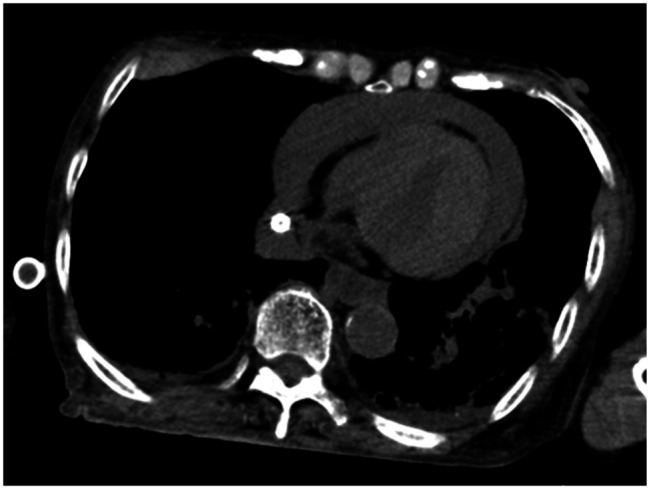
Axial chest computed tomography (CT) image on admission. Imaging revealed a prominent pericardial effusion and fractures of the sternum and multiple ribs, without evidence of pneumothorax or haemothorax
